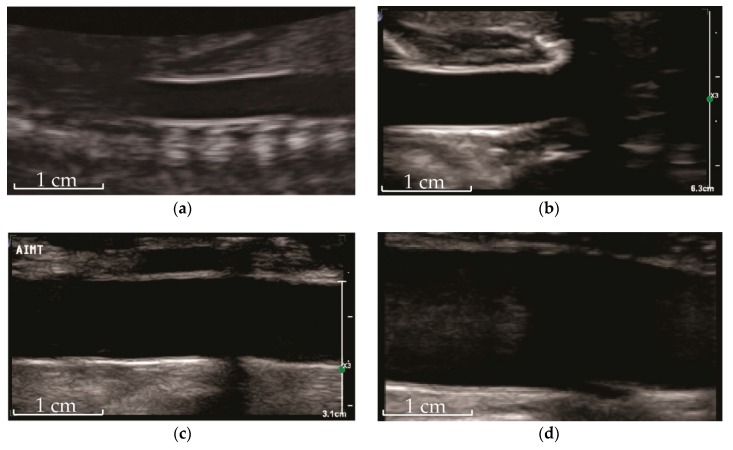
Ultrasound of abdominal aorta showing intima-media complex in a (a) fetus, (b) newborn, (c) child (8 years), and (d) young adult (20 years). Indicative scale as indicated.
